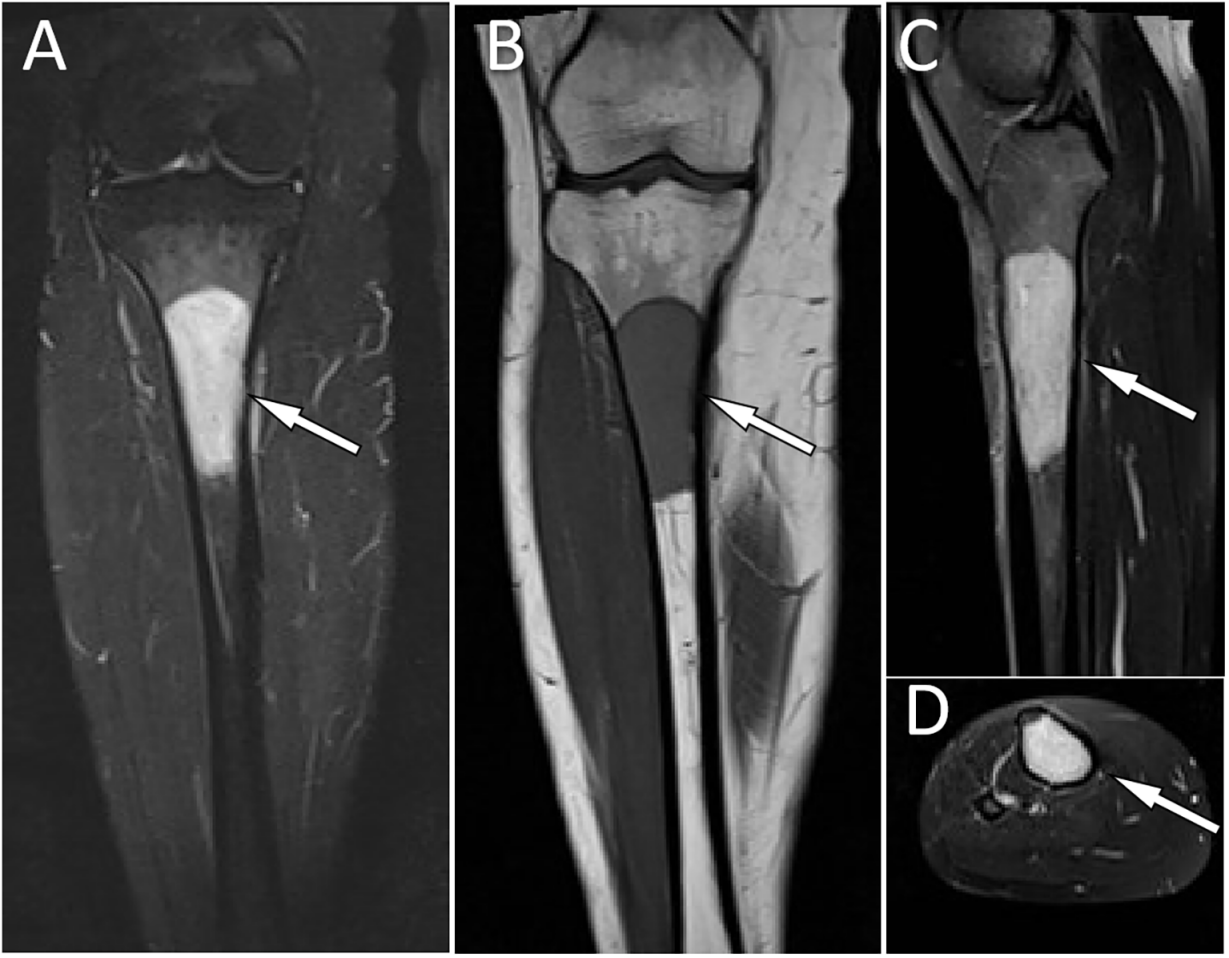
MRI showed a homogeneous signal of the lesion, with hypersignal on T2WI (A) and hyposignal on T1WI (B). Contrast-enhanced T1WI showed significant homogeneous enhancement (C), coronal and (D), axial.
